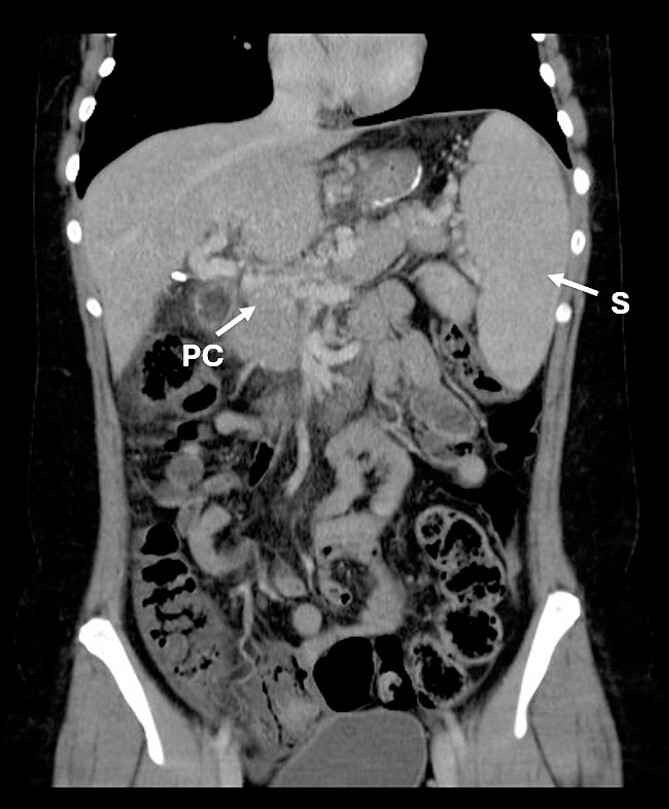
CT scan showing portal cavernomatosis and massive splenomegaly. PC, portal cavernomatosis; S, splenomegaly.
